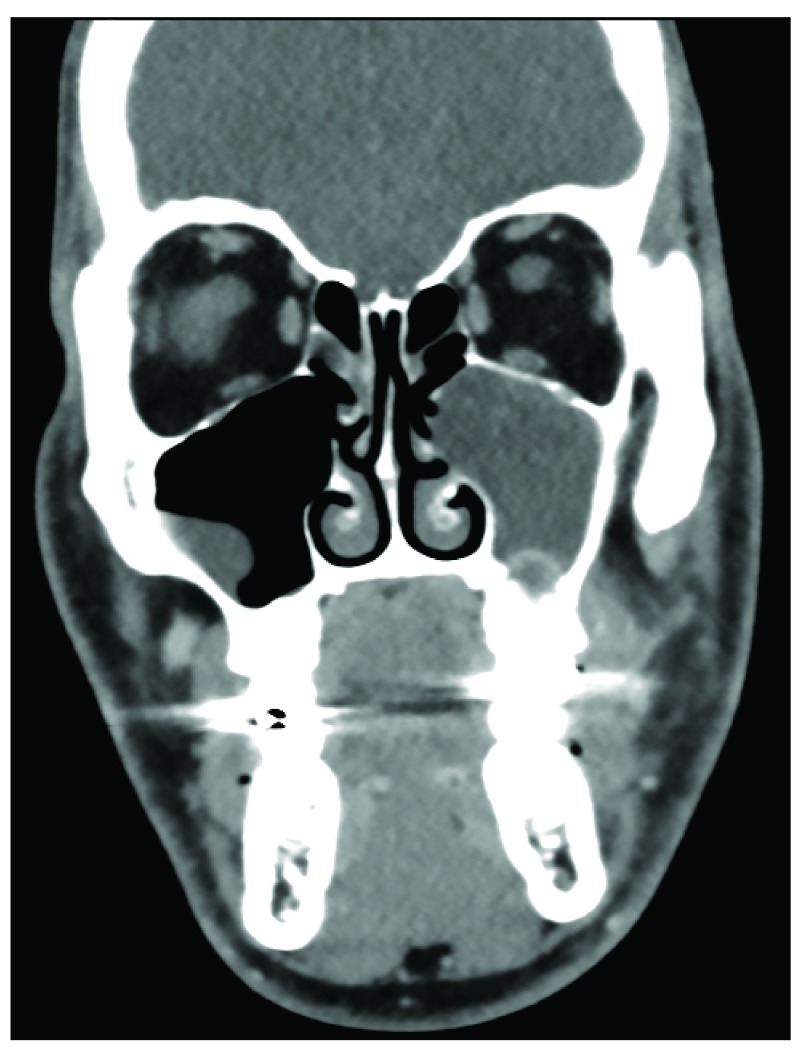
Odontogenic sinusitis. Coronal CT scan demonstrating a periapical lucency extending from tooth #14, resulting in localized left maxillary odontogenic sinusitis.
